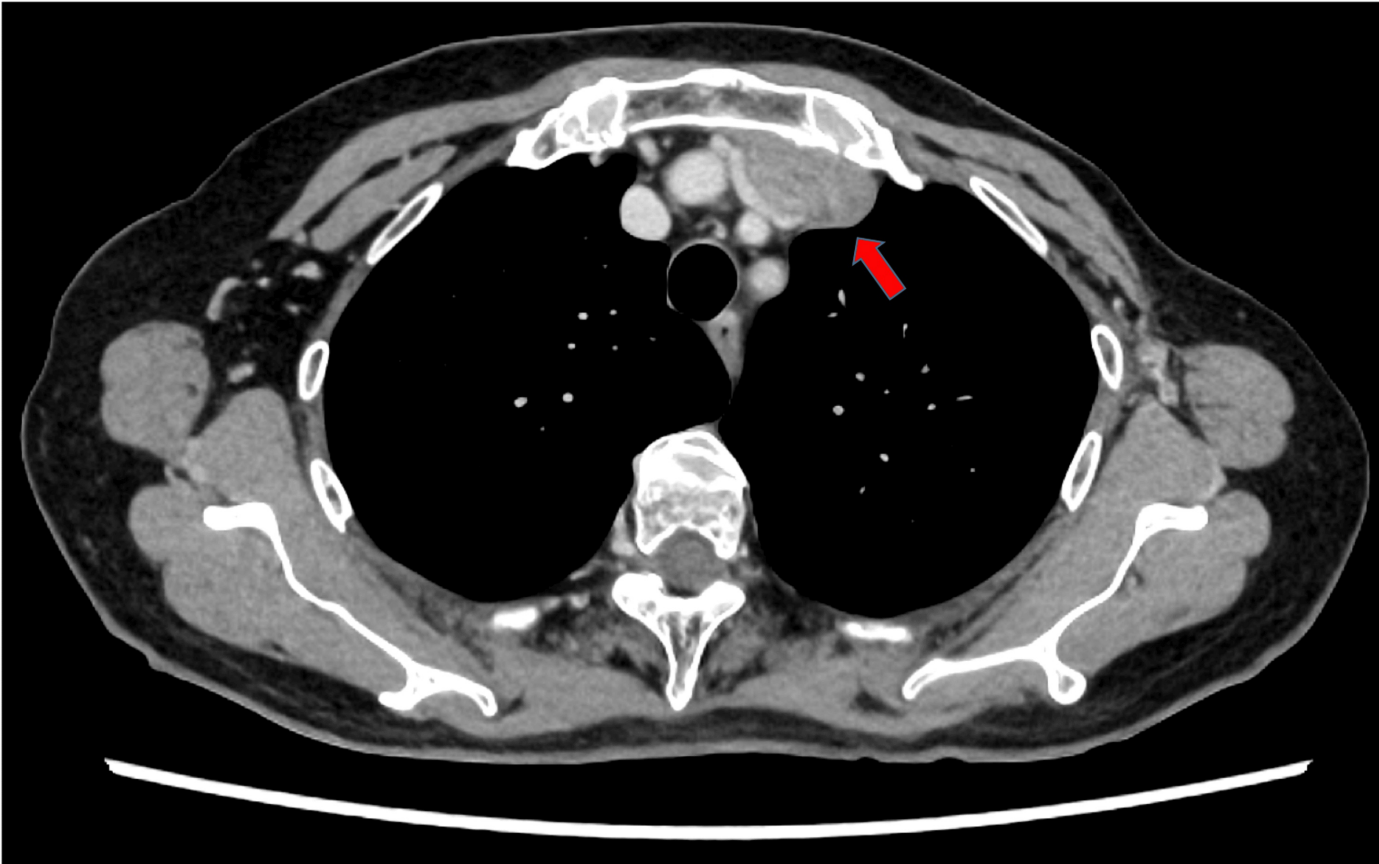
Mediastinal mass with vascular invasion on preoperative CT A mediastinal tumor (44x40x37mm) with invading the left innominate vein (red arrow).
